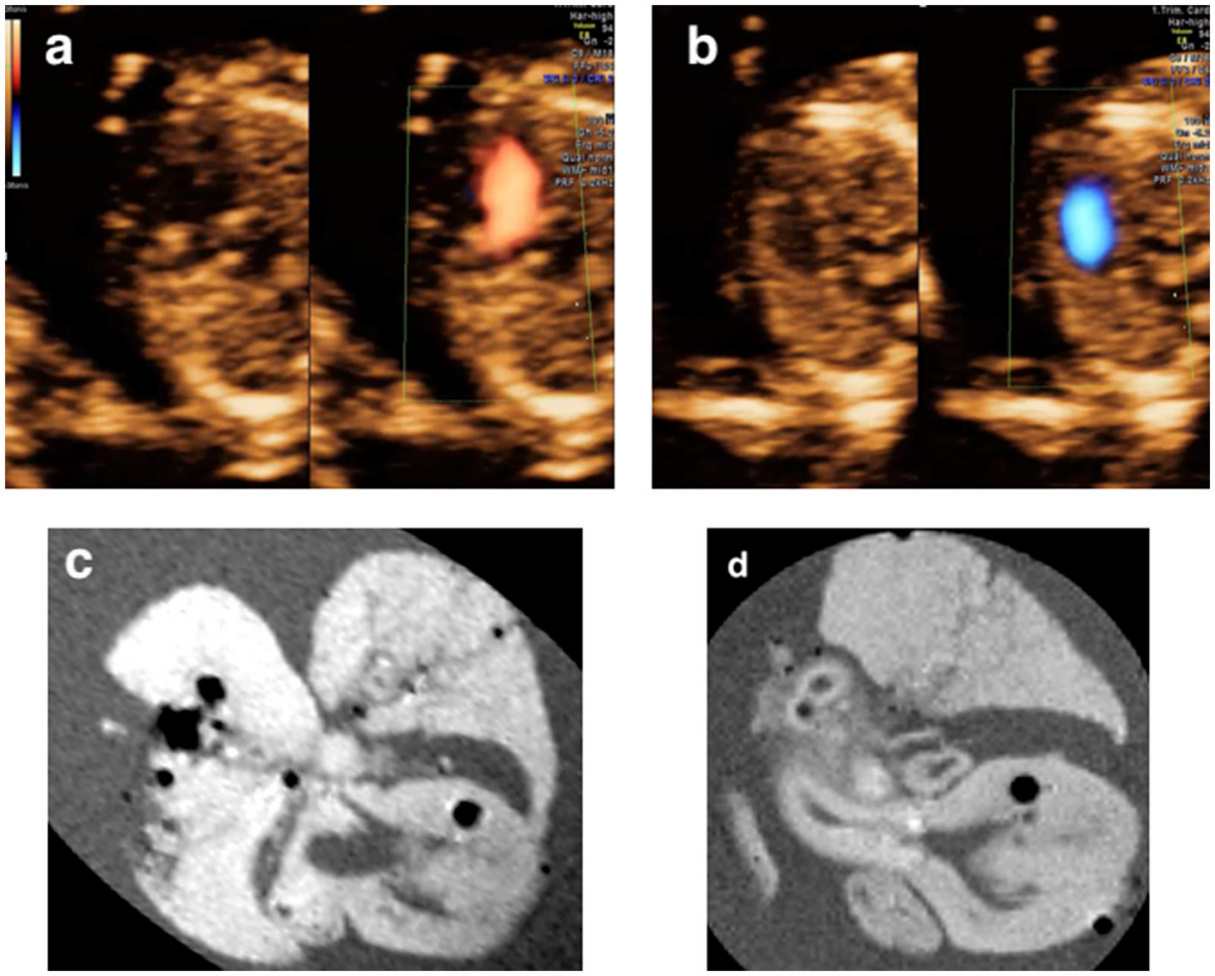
(a,b) A fetus terminated at 13+5 weeks of gestation and diagnosed as AVSD and suspected of great vessels malformation (Case 2 in Table 2); (c,d) Post-mortem MRI showing the AVSD at the four-chamber view and an overriding aorta at left ventricle outflow tract view.
